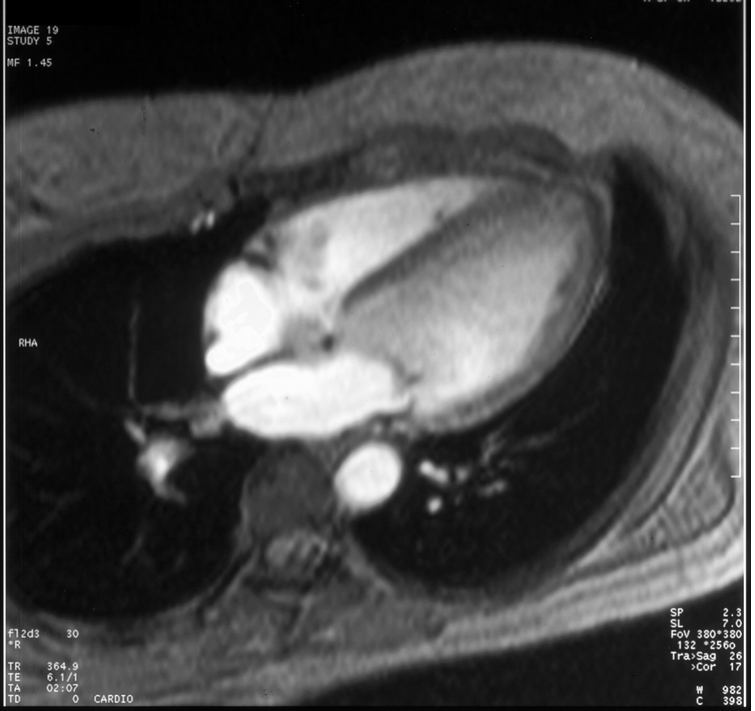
MRI cine turbo-flash images acquired on a 1.5 T scanner(Magnetom Vision-Siemens) in four-chamber (3A) and transverse plane, short-axis view (Fig. 4). These images didn't show congenital heart disease.
